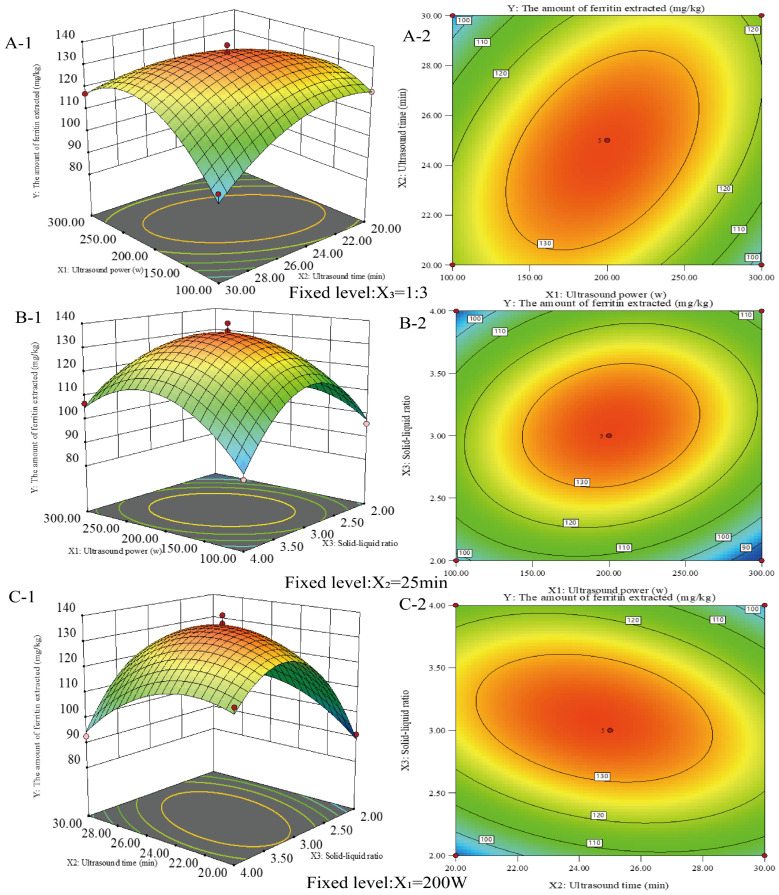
Response surface plots (A-1, B-1, and C-1) and contour plots (A-2, B-2, and C-2) showing the interaction effect of ultrasonic power (X_1_), ultrasonic time (X_2_) and solid–liquid ratio (X_3_) on the amount of ferritin extracted from the livers of the northern pike with ultrasonic-assisted extraction.
